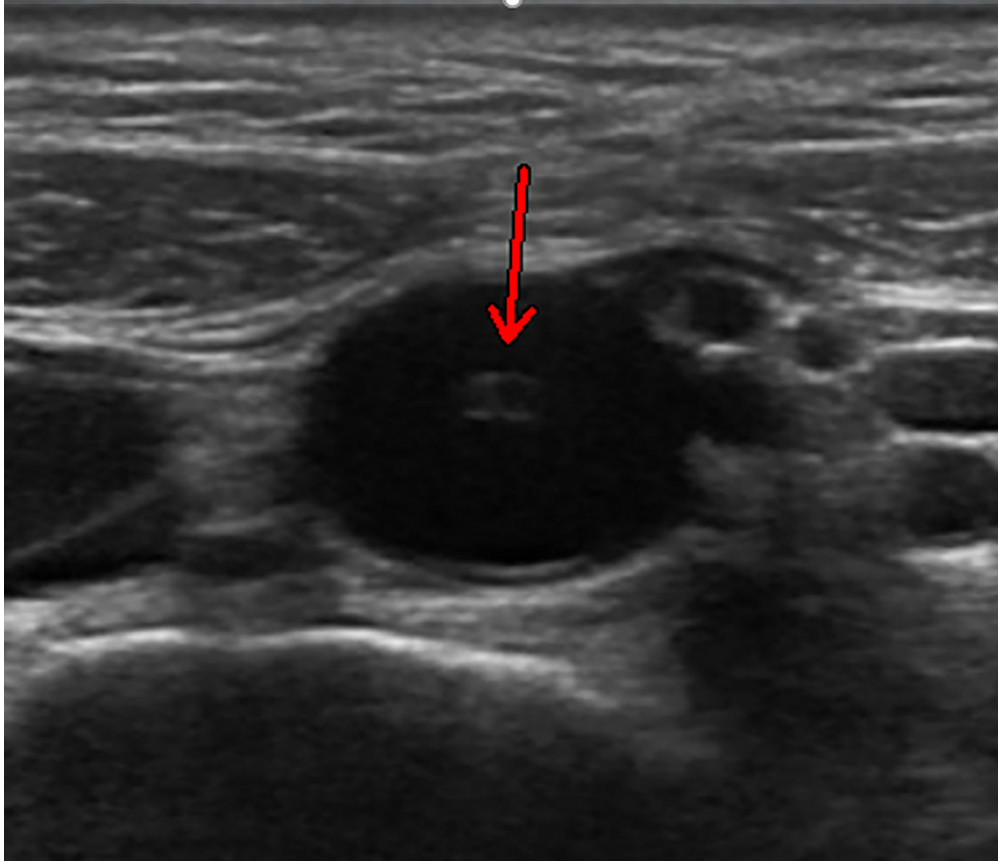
Transverse formal ultrasonographic view of an echogenic tubular structure (arrow) in the aorta.
